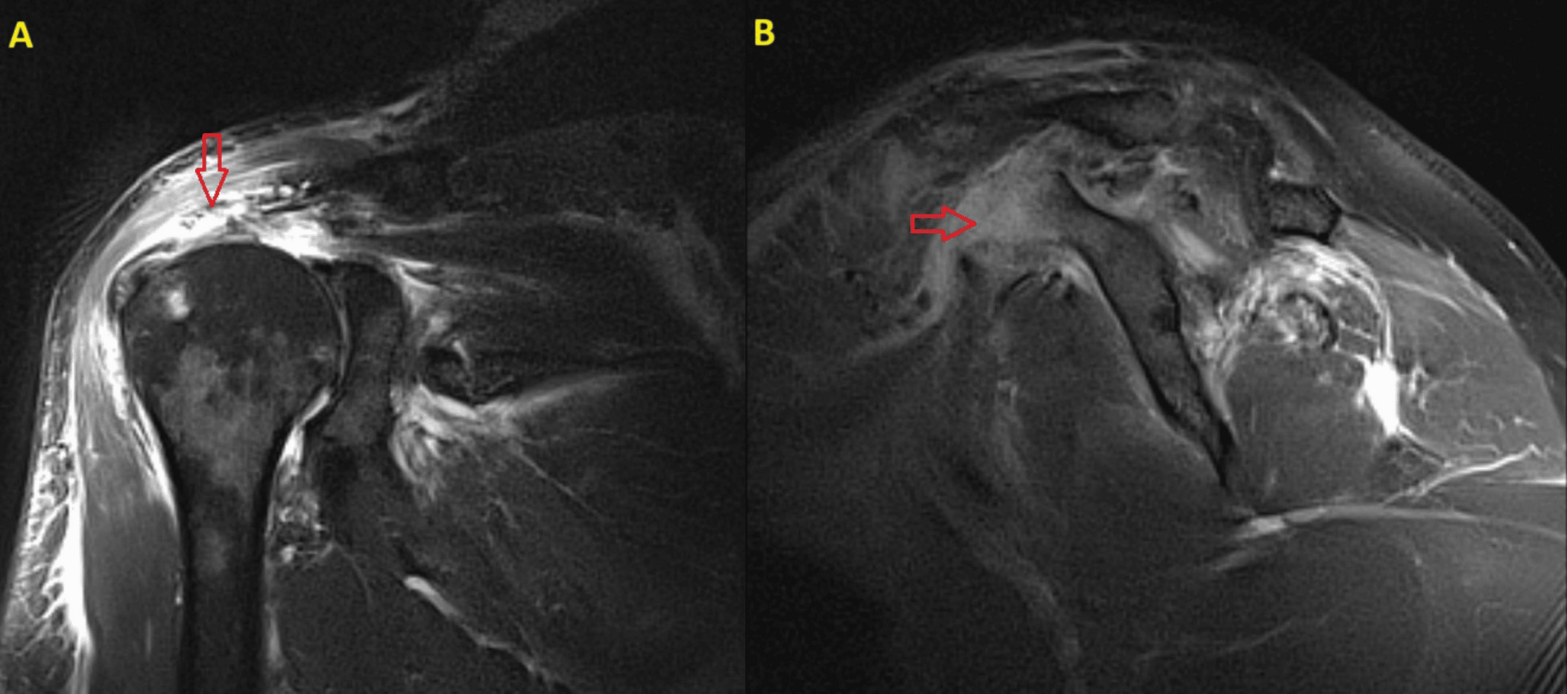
Preoperative MRI. (A) Coronal view: The red arrow indicates the rotator cuff tear. (B) Sagittal view: The red arrow demonstrates the coracoid process fracture.
